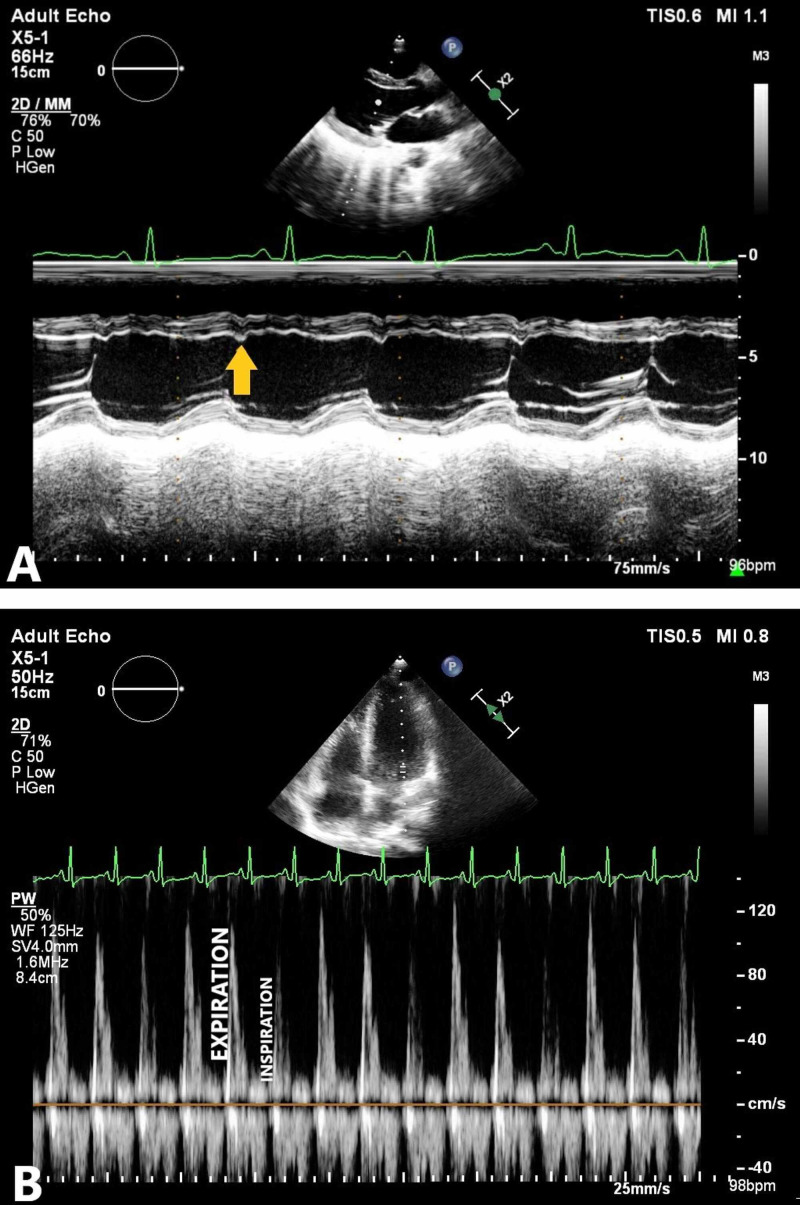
A) M-mode echocardiogram recording showing abnormal motion of the ventricular septum with notching (yellow arrow) in the early diastole, representing the diastolic dip. B) Pulsed-wave doppler spectrum of mitral inflow velocities demonstrating marked respiratory variation (more than 25%) of peak E-wave velocity.
